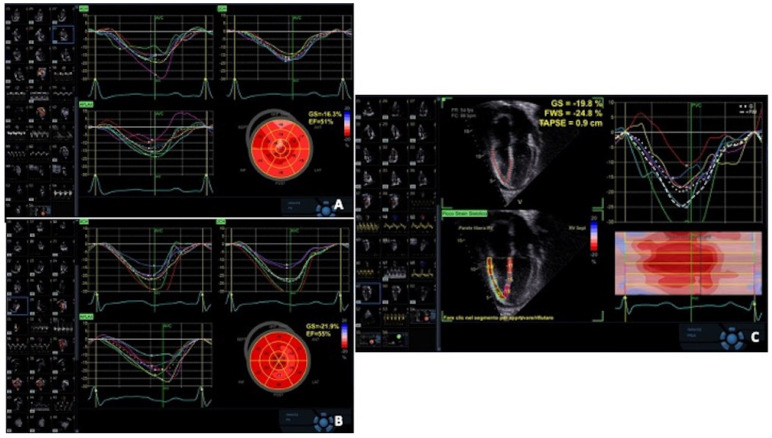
Global longitudinal strain (GLS) measured by speckle tracking echocardiography showing a reduced GLS for the left ventricle (3R—acute cellular rejection was confirmed by endomyocardial biopsy) (A); a normal GLS for the left ventricle (acute cellular rejection resolution confirmed by endomyocardial biopsy) (B); a reduced GLS for the right ventricle (3R—acute cellular rejection was confirmed by endomyocardial biopsy) (C).
